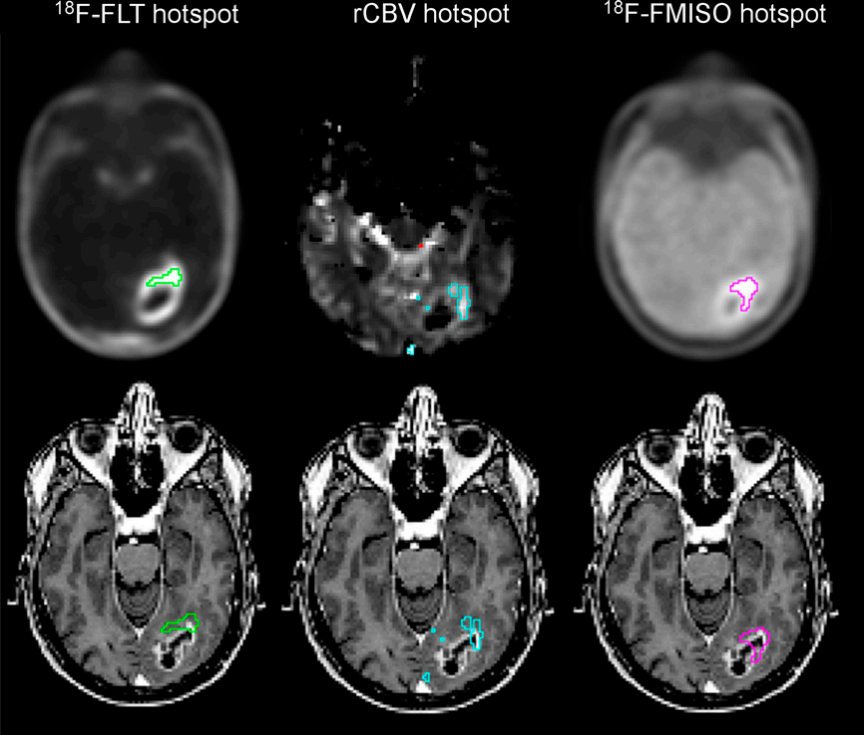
Example of proliferative hot spot in green, hypervascularized hot spot in blue, and hypoxic hot spot in pink on segmented (top) and overlaid (bottom) T1w-Gd MR images.
